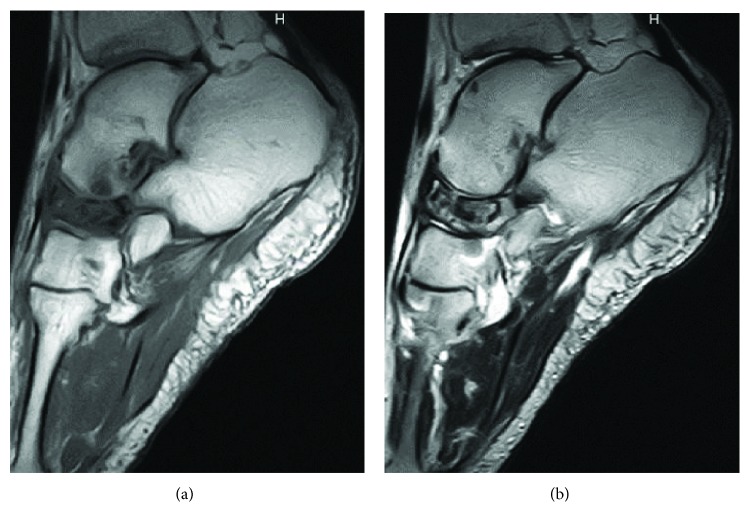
MRI shows low signal-intensity areas on T1-weighted images (a) and T2-weighted images (b) in the marrow of the tarsal navicular.
